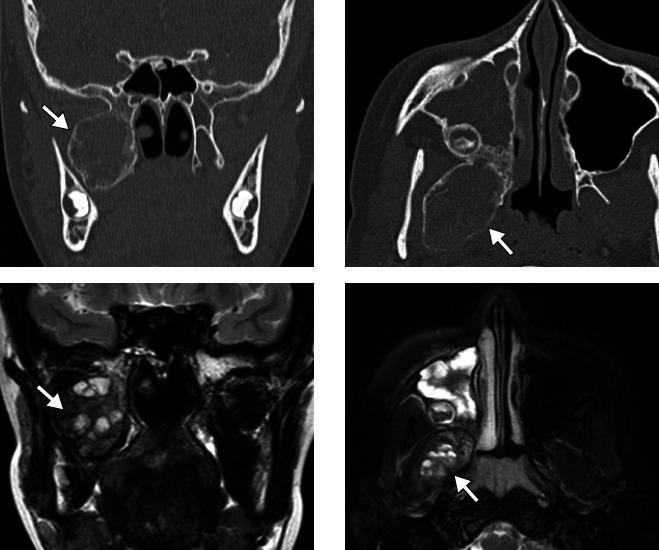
Aneurysmal bone cyst in a 13-year-old male patient. MRI scans showing
